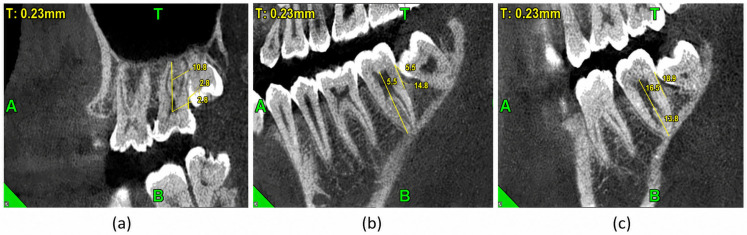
Representative cone-beam computed tomography (CBCT) images of permanent molars with taurodontism, classified according to the taurodontic index (TI). From left to right: (a) hypotaurodontism (TI = 25.92%), (b) mesotaurodontism (TI = 37.16%), and (c) hypertaurodontism (TI = 47.10%). Yellow lines and numeric values indicate the linear measurements used to calculate the TI. The green letters are orientation markers generated by the CBCT viewing software.
